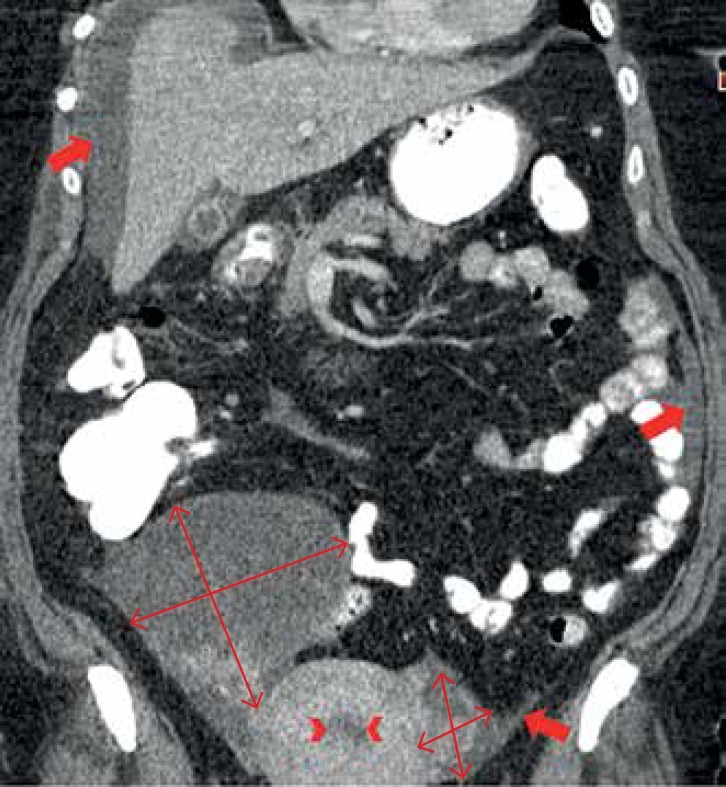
Thoracoabdominopelvic contrast-enhanced CT scan (coronal reconstruction
